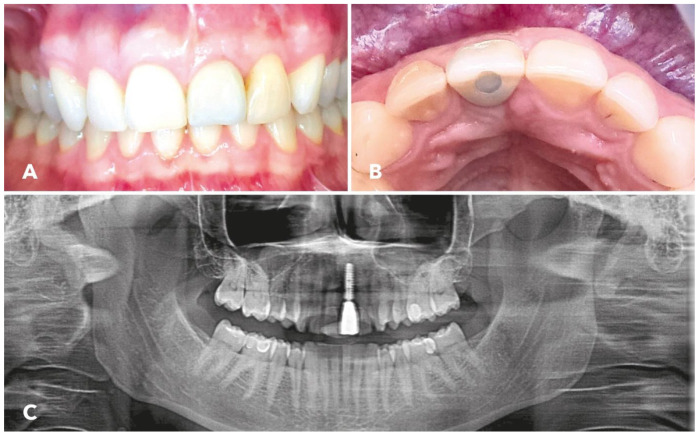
(A) Frontal view 1 year after the prosthetic restoration of implant 21; the color of crown 21 is slightly darker compared to 11; the papilla distal to implant 21 has been reconstructed. (B) View after prosthetic rehabilitation with slight defect of the buccal outer contour. (C) Panoramic radiograph 3 years after prosthetic rehabilitation.
